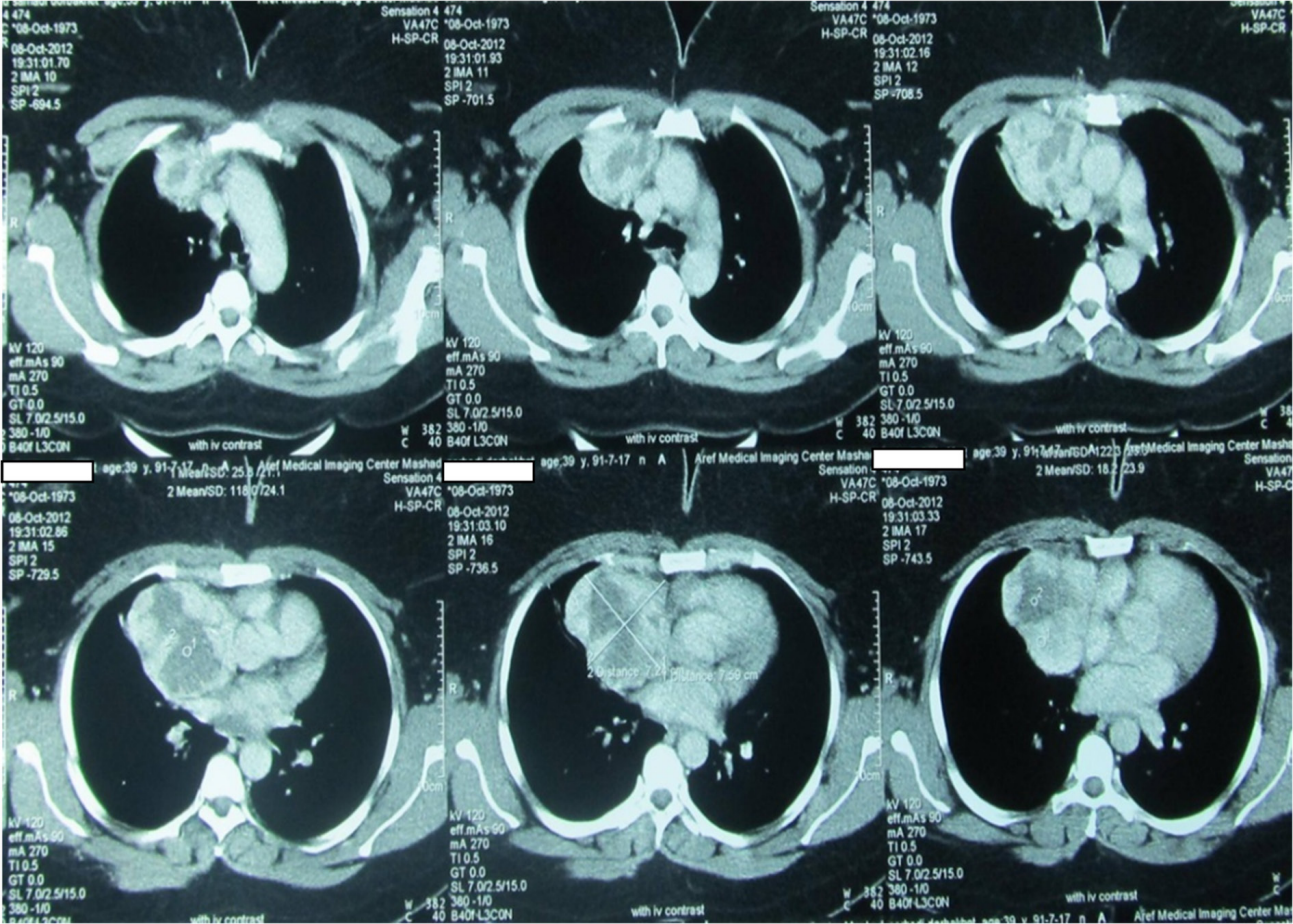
Multislices thoracic CT in axial slices shows mediastinal mass extending from suprasternal notch to above liver.
